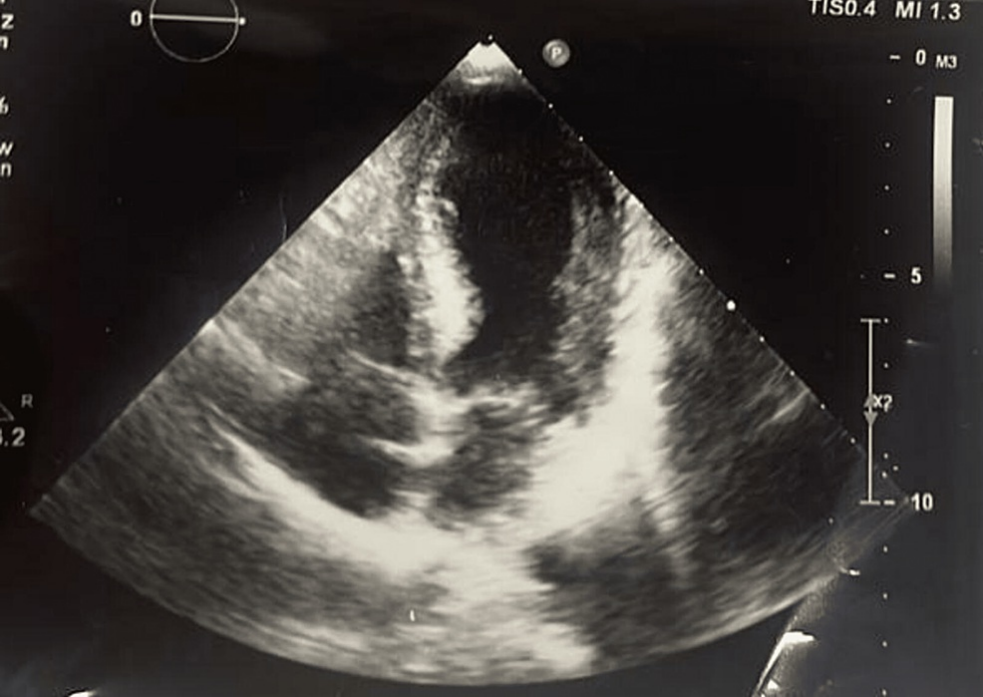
Transthoracic echocardiography showing apical ballooning
